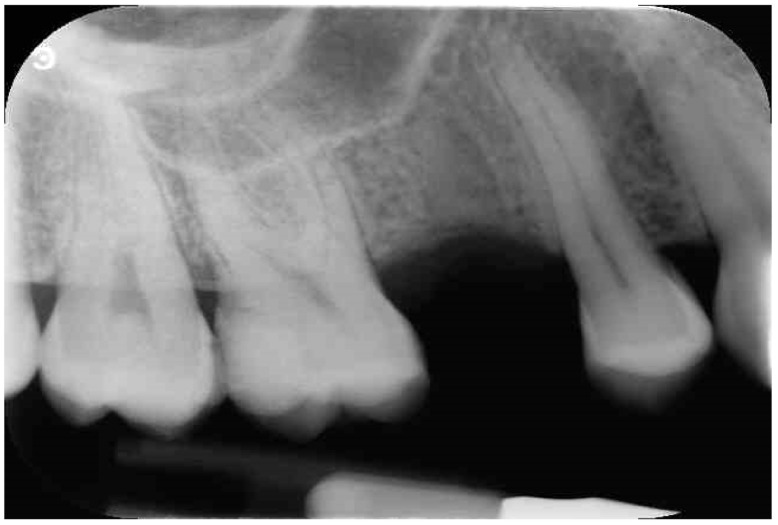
Preoperative periapical X-ray.
